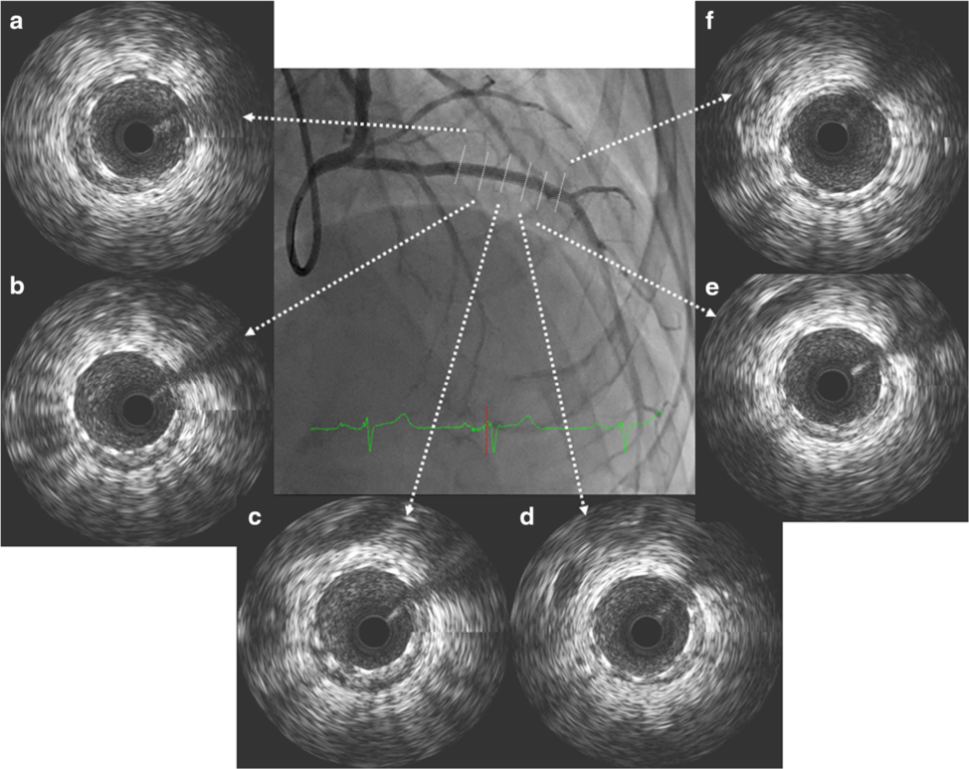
Intravascular ultrasound image demonstrating that the honeycomb-like structure in the mid left anterior descending coronary artery had been compressed by stent implantation (a–f)
